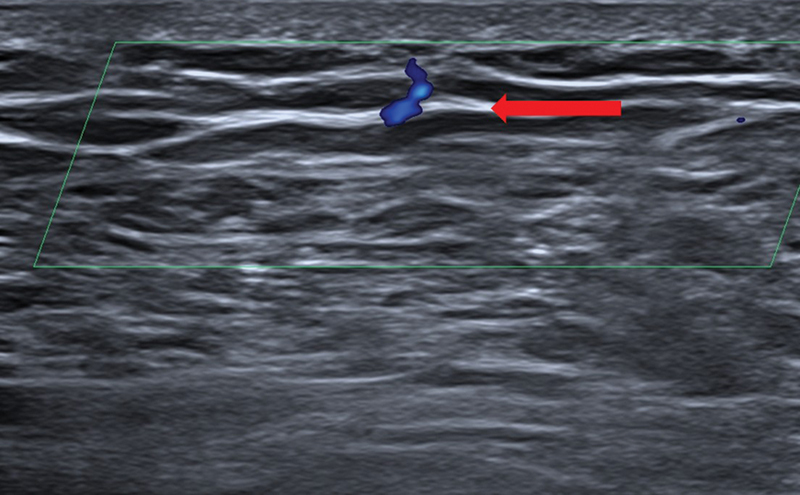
Color Doppler ultrasonography. A linear 24 MHz probe was used. The red arrow shows the superficial fascia. Here, the perforator is piercing the superficial fascia.
